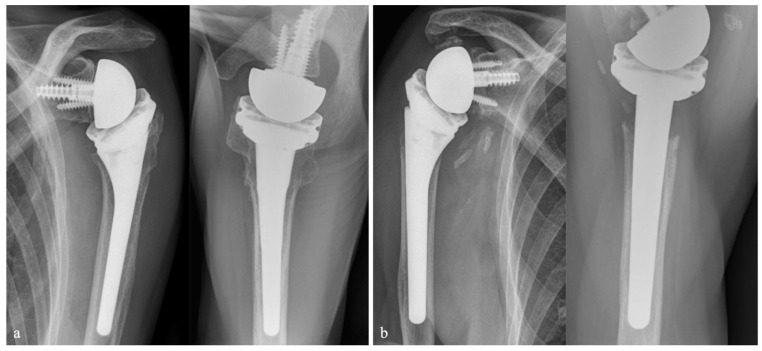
Radiographs at follow-up, (a) anatomic GT and LT healing in AP and axial view, (b) GT migration with nonunion, ossifications and scapular notching in AP view, GT and LT resorption in axial view (GT: greater tuberosity, LT: lesser tuberosity, AP: anterior–posterior).
